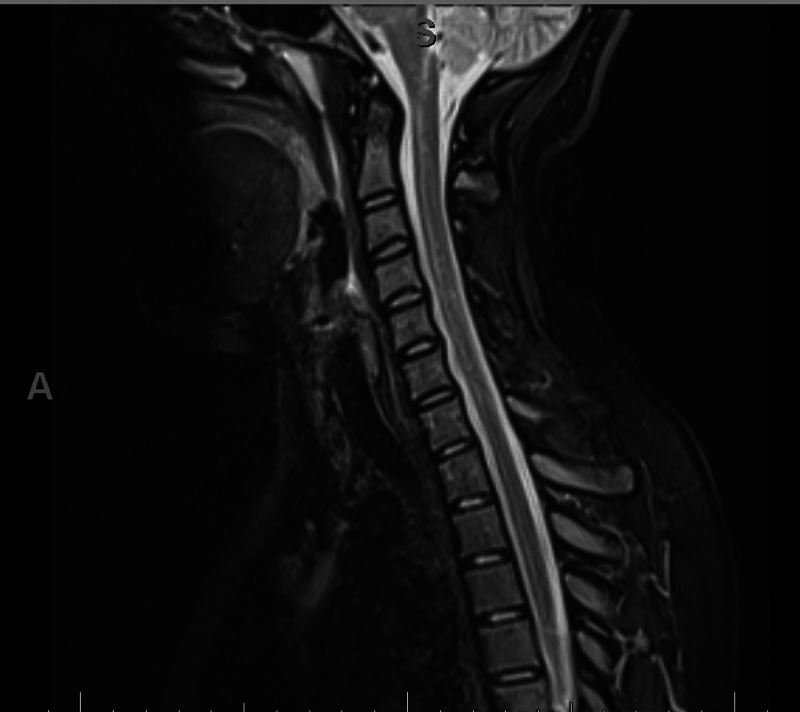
MRI cervical spine without contrast
